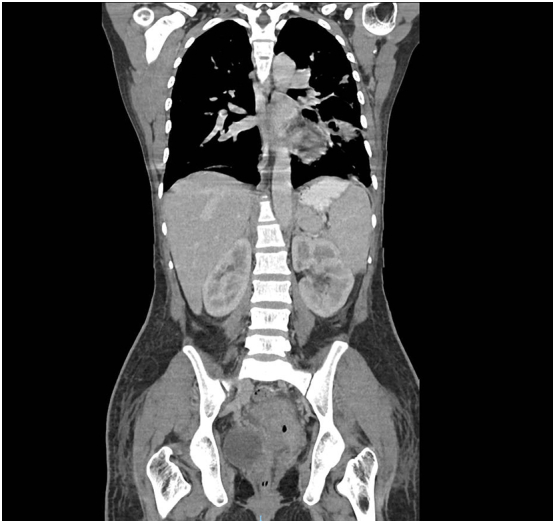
A CT scan demonstrating central cavitation in the superior segment of the left lower lung suggests a septic pulmonary embolism.
